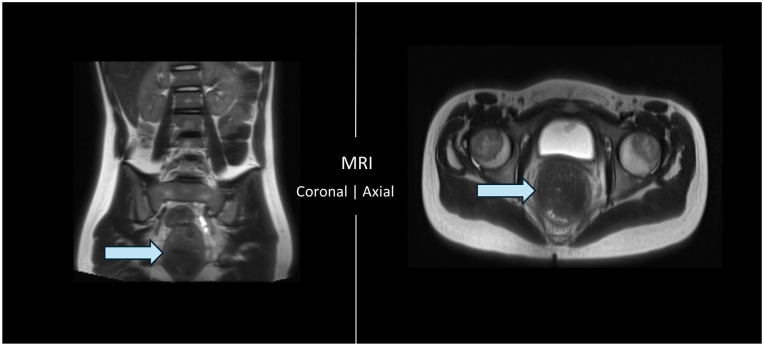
MRI of the abdomen without contrast from the coronal (L) and axial (R) perspectives showing dilated bowel loops and impacted rectum. Arrows point to fecal impaction in both perspectives. MRI: magnetic resonance imaging.
